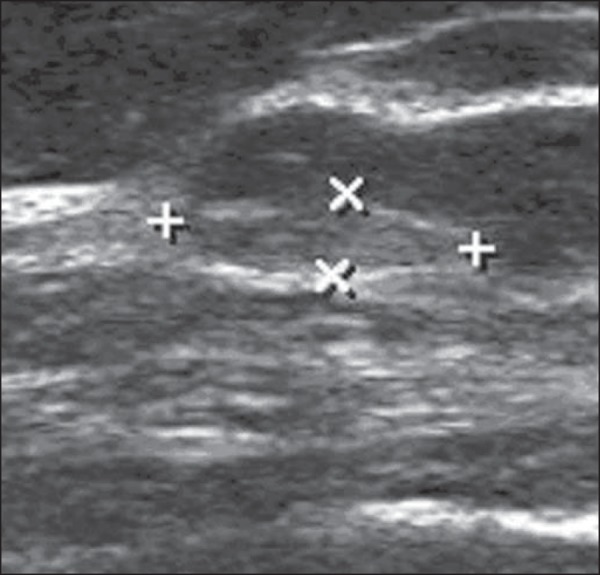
Fibroadenoma*. Follow-up ultrasonography showing
